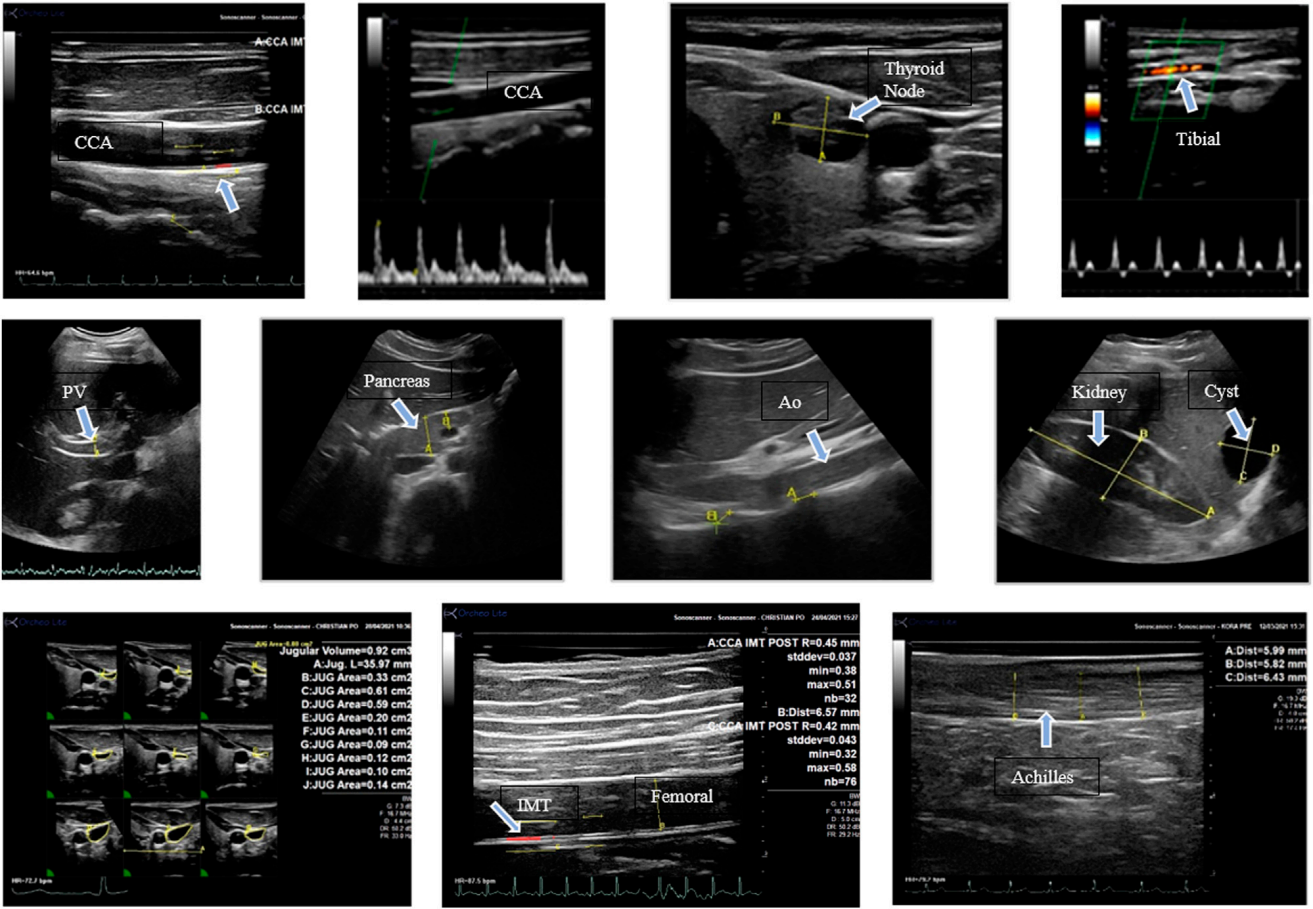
Images with arrows indicating various structures assessed in this study. The images are described from left to right. Top row: longitudinal view of the common carotid artery; (CC) carotid Doppler velocity; thyroid with a nodule; tibial artery Doppler velocity. Middle row: portal vein (A); head of the pancreas; abdominal aorta (Ao) and lumbar vertebral distance; kidney (A-B) and liver cyst (C-D). Bottom row: jugular vein volume measurements from the 3D reconstruction, femoral artery IMT, Achilles tendon measurement.
